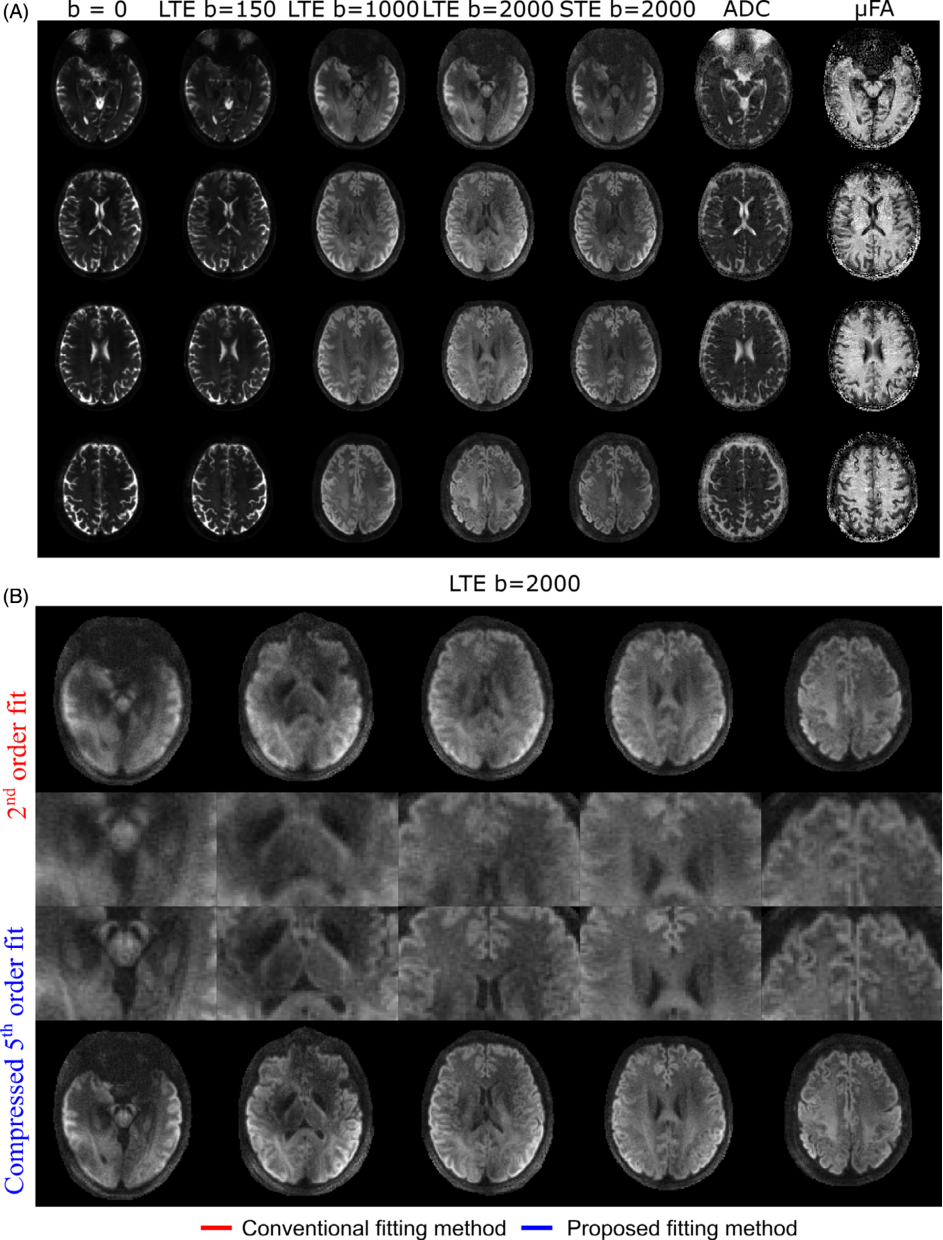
(A) Mean sample image slices reconstructed for the linear and spherical tensor‐encoded acquisitions incorporated in the b‐tensor encoding scan, as well as computed ADC and μFA maps. Reconstructions were informed with compressed fifth order field dynamics, using the compression matrix determined from “scan 1” calibration data. (B) Comparison of mean DWI from the linear tensor‐encoded (LTE) scan (*b* = 2000 s/mm^2^) for reconstructions informed with conventional second order fits (top) and compressed fifth order fits (bottom), with zoom‐ins highlighting the blurring reduction observed when implementing compressed fifth order fits.
